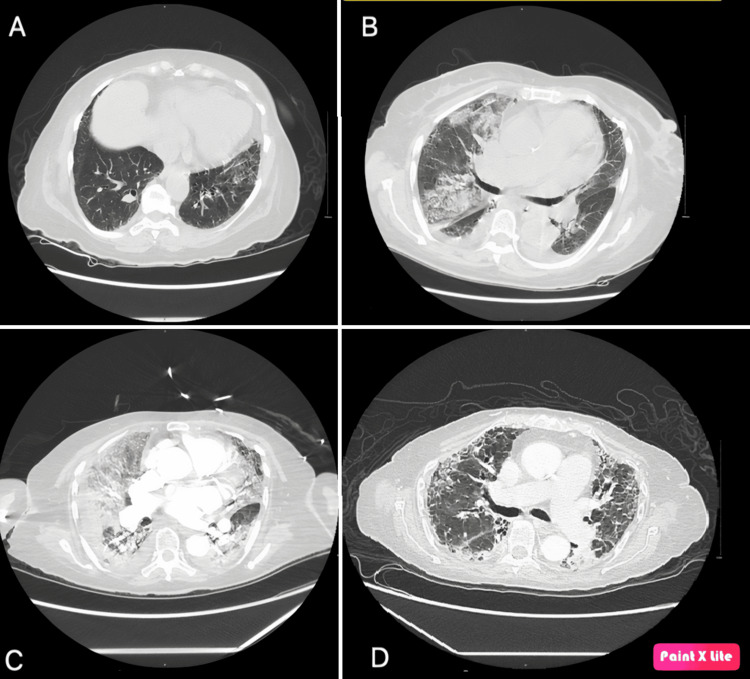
Imaging timeline of interstitial lung disease complicated by COVID-19 pneumonia and subsequent recovery. (A) Interstitial lung disease identified at the time of diagnosis. (B) Development of new patchy opacities and small bilateral pleural effusions with COVID-19 pneumonia. (C) Interval worsening of diffuse ground-glass opacities with more confluent areas of consolidation superimposed on interstitial lung disease. (D) Follow-up chest CT three months later with interstitial lung disease and interval resolution of diffuse ground-glass opacities and consolidation.
